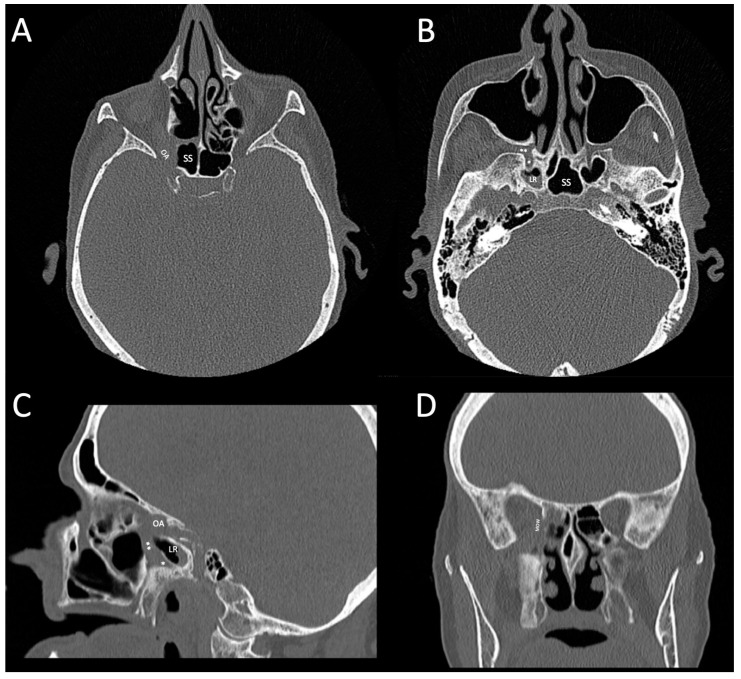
Initial CT prior to the first surgical intervention at the regional secondary otorhinolaryngology service showed sphenoid sinus opacification and the involvement of different adjacent structures. (A): axial CT shows the dehiscent lateral wall of the right sphenoid sinus (SS) and medial orbital apex (OA); (B): axial CT shows widened right Vidian canal (*), pterygopalatine fossa () and mucosal thickening in the lateral recess (LR) of the sphenoid sinus; (C): sagittal CT shows eroded superior part of right Vidian canal and mucosal thickening of the lateral recess of sphenoid sinus; (D): coronal CT shows mucosal thickening in the right posterior ethmoid sinus and dehiscence of medial orbital wall.
